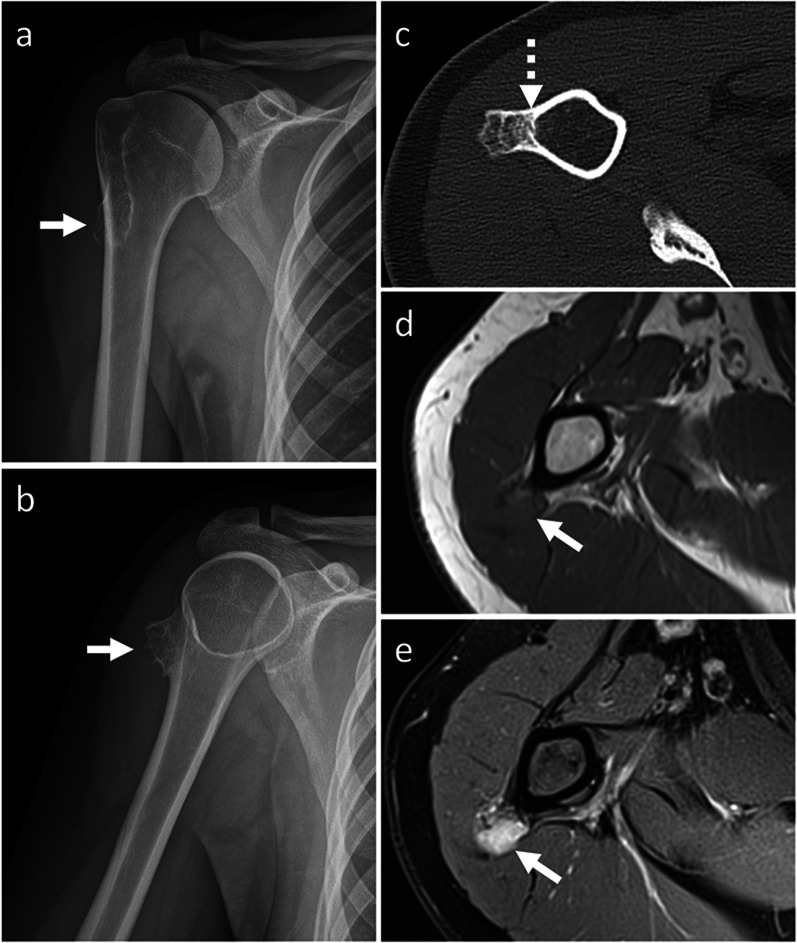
Periosteal chondrosarcoma. On X-rays (a, b), a mineralized surface-based mass of the proximal humerus is seen. On axial CT image (c), the mass is cortex-based and partially ossified. Cortical remodeling and erosion (dashed arrow) are noted. On axial T1-weighted (d) and proton density-weighted (e) MRI sequences, the mass shows low and high signal, respectively. No marrow or soft-tissue extension is noted. Periosteal chondrosarcoma was pathologically proven. White arrow points at the lesion in all images
